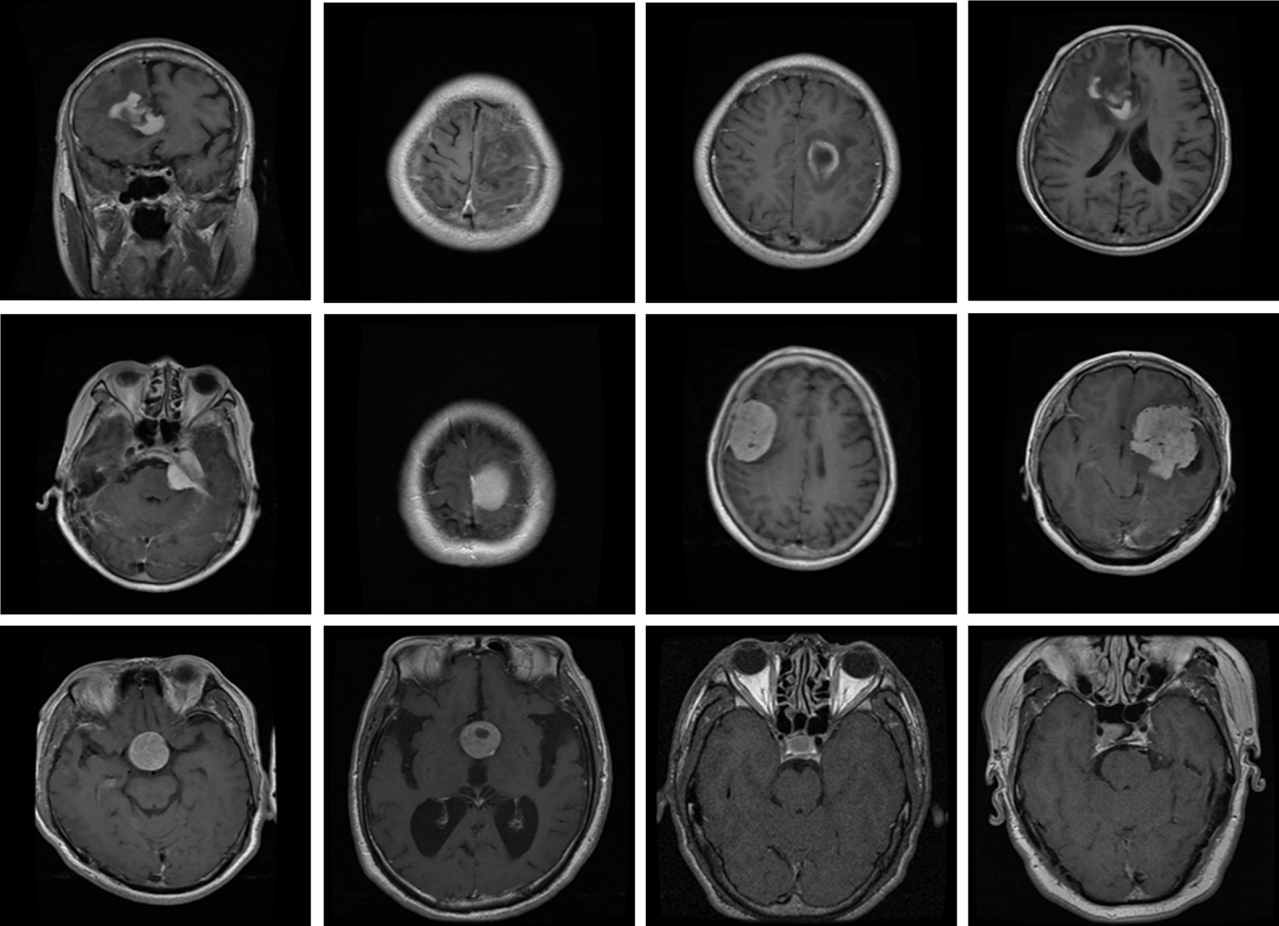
Images from the CE-MRI dataset, upper row: Glioma, middle row: Meningioma, lower row: Pituitary.
